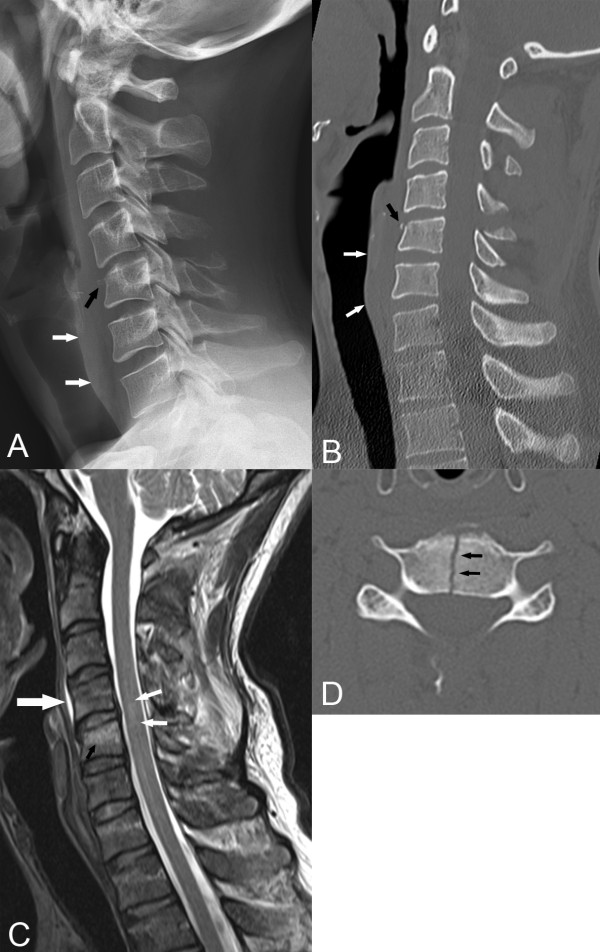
Cervical Spine Trauma. A. A lateral cervical spine radiograph reveals a C4 spinous process fracture with inferior displacement. The C5 vertebral body is slightly compressed with a tiny teardrop fragment anteriorly (black arrow). The prevertebral soft tissue margin is clearly visualized and there is a suggestion of widening secondary to edema. (white arrows) B. A sagittal reconstruction of a CT scan reveals the same findings as the radiograph but in much more detail. C. A sagittal T2-weighted MR image reveals high signal intensity in the C5 vertebral body (black arrow), in the posterior soft tissues, and within the cord itself. The latter finding is characteristic of spinal cord contusion, edema, and/or hemorrhage. D. An axial CT image shows a complete vertical fracture (arrows) through the C5 vertebral body, a finding not seen on the lateral radiograph or sagittal CT display. (Images courtesy of Lindsay J. Rowe, Newcastle, Australia).
